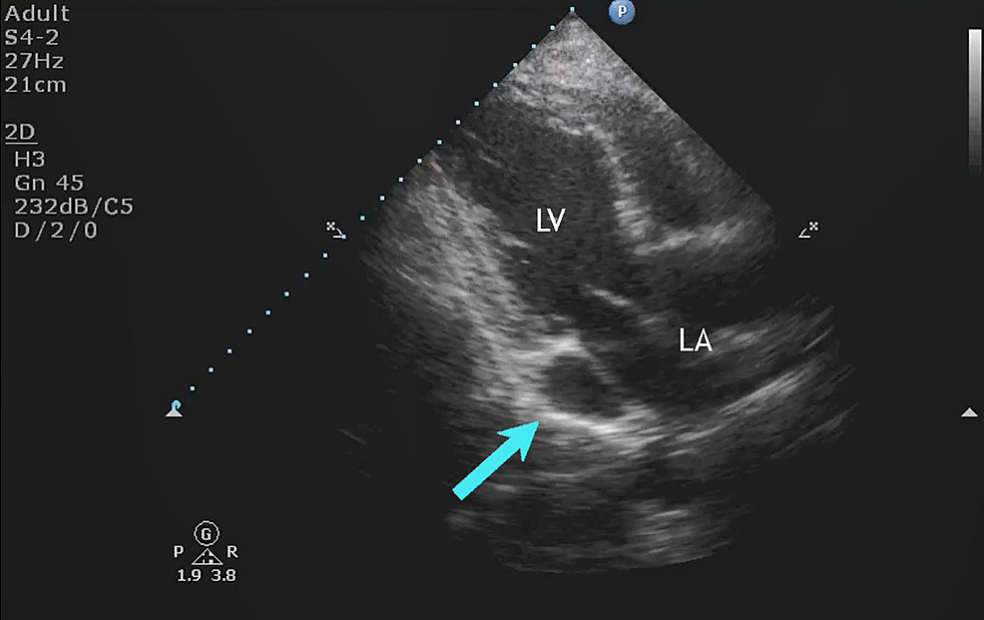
Echocardiogram showing the dilated coronary sinus. Echocardiogram showing the dilated coronary sinus (arrow). No associated congenital malformations were seen.
